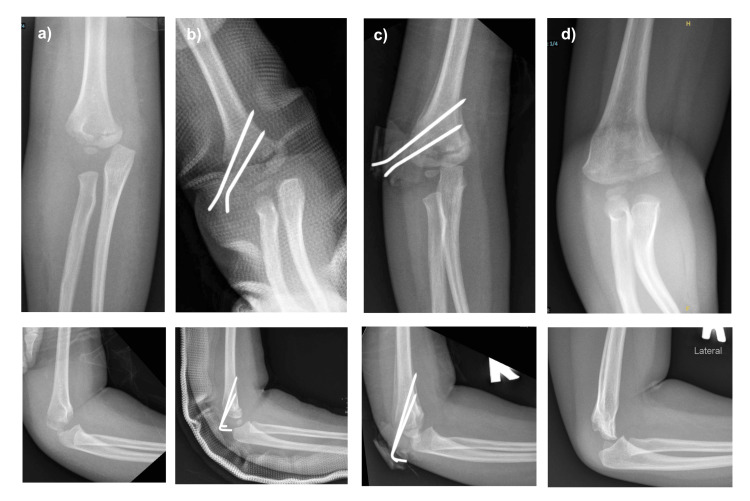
Serial elbow radiographs of case 2 Serial elbow radiographs at: (a) initial injury: showing posteromedial displacement of distal humerus epiphysis; (b) immediate post-operative radiograph following closed reduction and percutaneous pinning; (c) 3 weeks post-surgery: showing callus formation; (d) 4 months: showing a healed fracture with residual varus deformity on the coronal plane.
